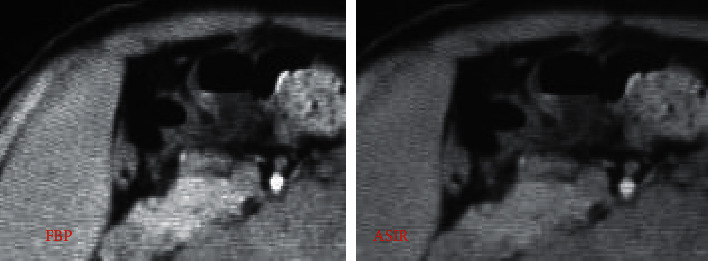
The partially enlarged views of liver imaging after reconstruction of the abdominal CT image of an ICP patient in two ways. *Note.* The average scores of subjective evaluations of FBP reconstructed images show image quality of 2.34 points, noise of 2.33 points, and artifacts of 4.45 points; the average scores of subjective evaluations of ASIR reconstructed images show image quality of 3.9 points, noise of 1.85 points, and artifacts of 2.34 points.
